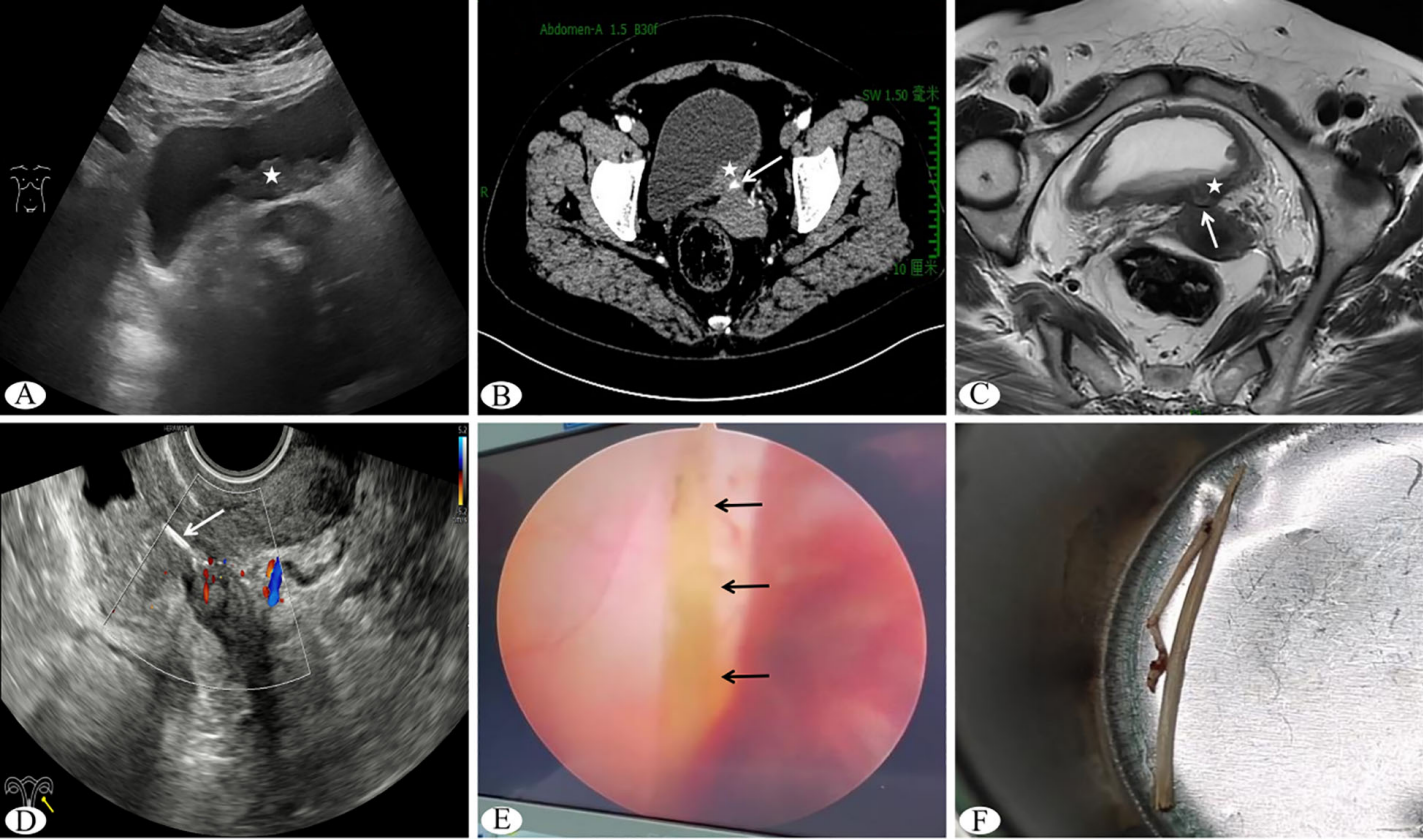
(A) Transabdominal ultrasound showing a posterior bladder wall mass (indicated by pentagram); (B) Contrast-enhanced CT demonstrating posterior bladder wall mass (pentagram) and linear dense shadow (arrow); (C) Contrast-enhanced MRI showing posterior bladder wall mass (pentagram) and linear hypointense signal (arrow); (D) Transvaginal ultrasound revealing a linear hyperechoic band in the uterovesical space (arrow); (E) Laparoscopic view of fish bone (arrow); (F) Extracted fish bone postoperatively, measuring approximately 3 cm in length.
